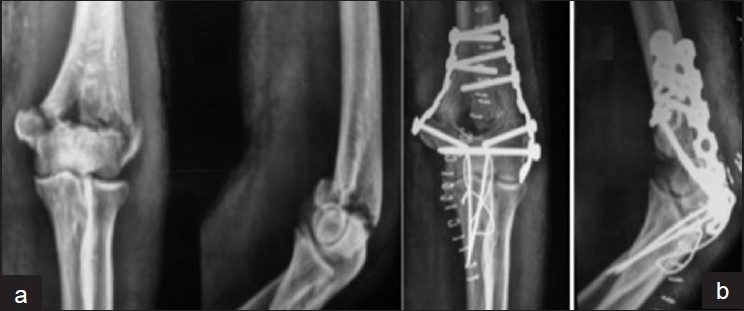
Radiograph showing C2 type fracture stabilized by, intercondyler cancellous screw and parallel plate fixation by contoured plates. Extraarticular osteotomy stabilsed by two parallel K-wires and tension band wire
